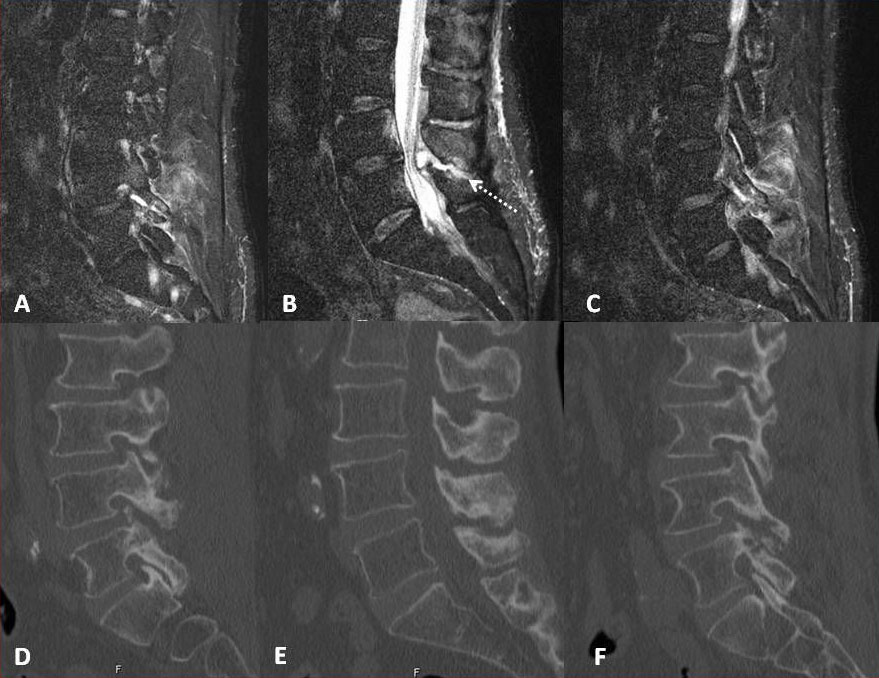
Right to left sagittal T2 MRI images with their associated CT sagittal reconstructions beneath, show the fluid-like (isodense and isointense to CSF) signal in the right greater than left facet joints, as well as diffusely increased signal in the adjacent paravertebral muscles. (2A and C) The midline sagittal MRI image shows the compressive epidural portion of the cyst, with a stalk that trails into the interspinous space (dotted line), where it communicates with the facet cysts. (2B) The CT images show non-specific chronic destructive changes to the L4/5 facet, typical of uncomplicated lumbar facet cysts. (2D, E and F)
